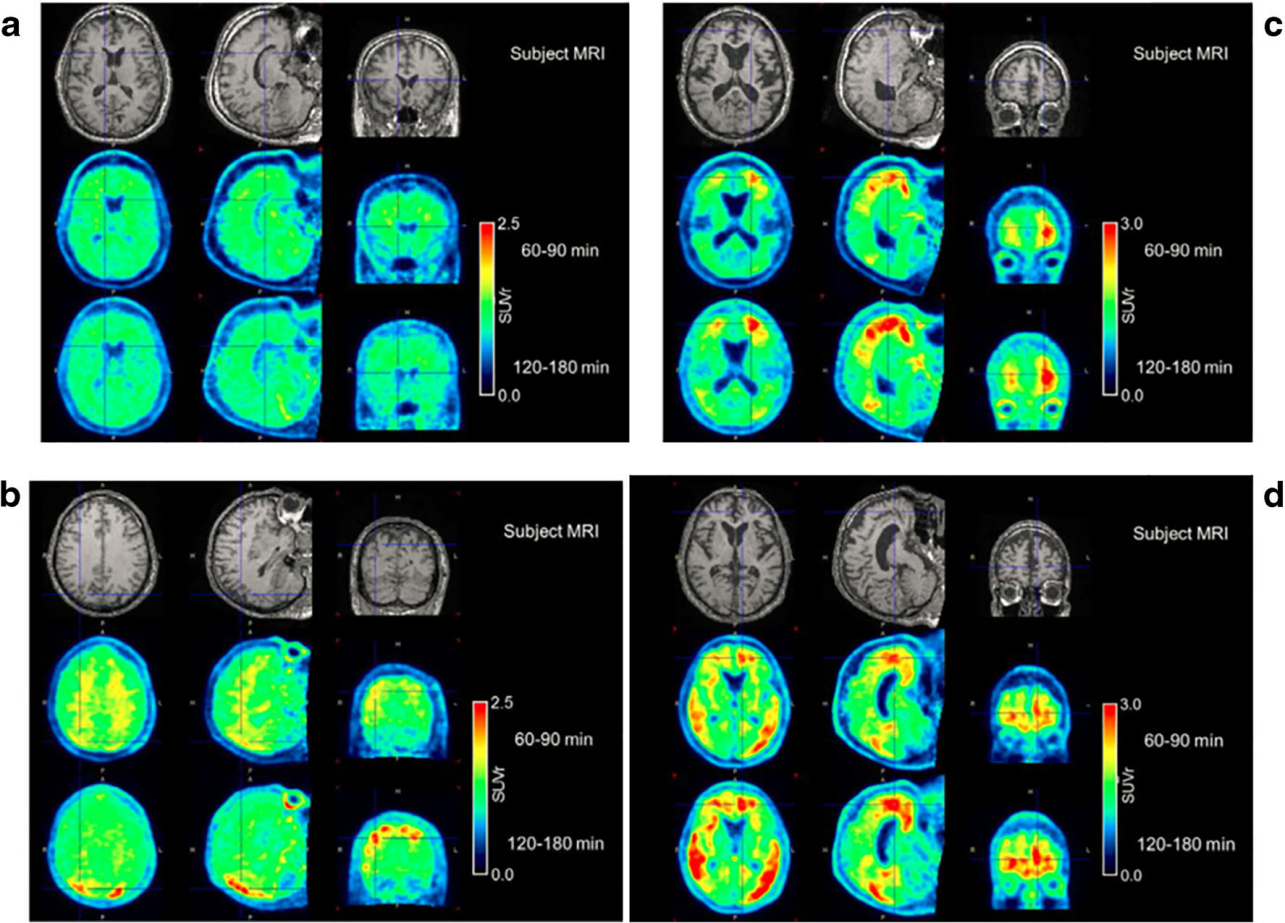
Individual MRI (top), 60–90 min (middle), and 120–180 min (bottom). PET SUVr images from HV Subjects 13 (a), and 10 (b), and AD subjects 6 (c) and 11 (d). Signal in the occipital cortex in subject 10 underlie the location of pellets in the skull from a shotgun accident as a youth
